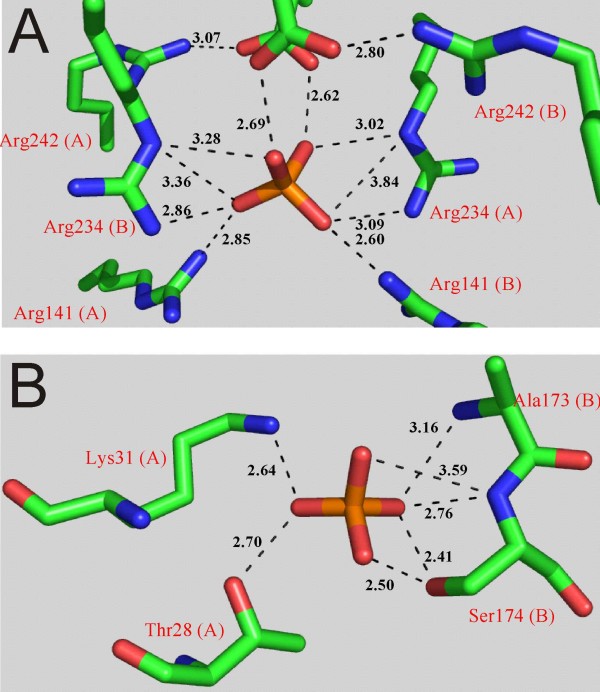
Close-up structures of the orthophosphate binding sites at the dimer interface of *Cc*GlgP. Panels A (Tower helix region; P-site) and B (CAP region) are drawn using the x-ray structure of *Cc*GlgP (PDB-accession code 2C4M). There are two identical CAP sites at the subunit-subunit interface, related by the internal symmetry of the enzyme dimer. H-bond distances are in Å, and coordinating residues contributed from subunit A or B are indicated in brackets.
